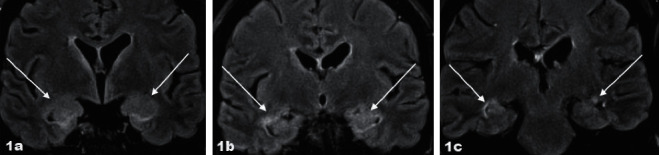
(a–c) Coronal FLAIR-MRI scan reveals slight enlargement and increased signal intensity of the amygdala and hippocampus bilaterally.
